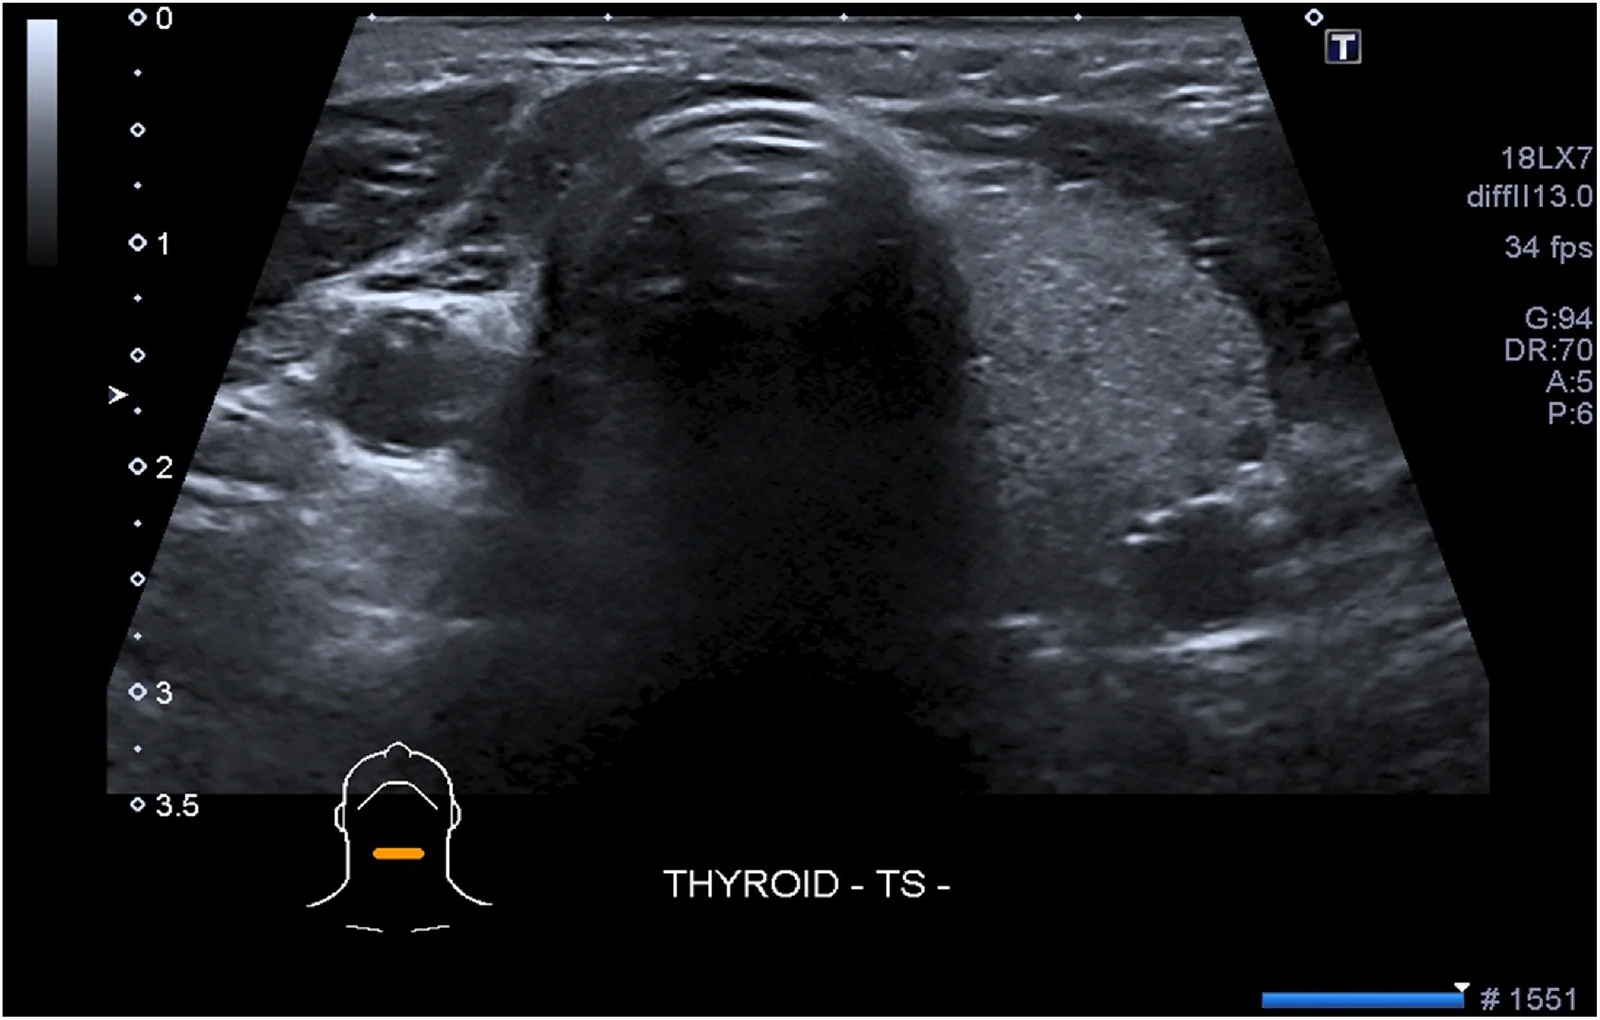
Thyroid ultrasound identifies a normal left lobe of thyroid with no focal lesion or suspicious malignant features. Previous right hemithyroidectomy with normal surgical bed.
